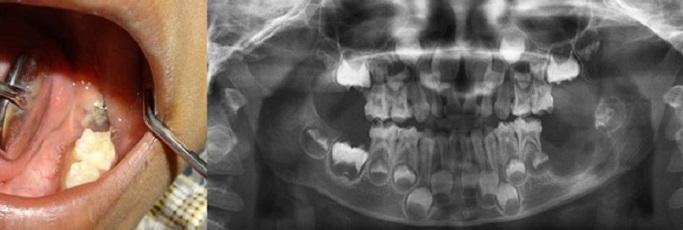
14^th^ day post-operative clinical picture and radiograph (OPG)
